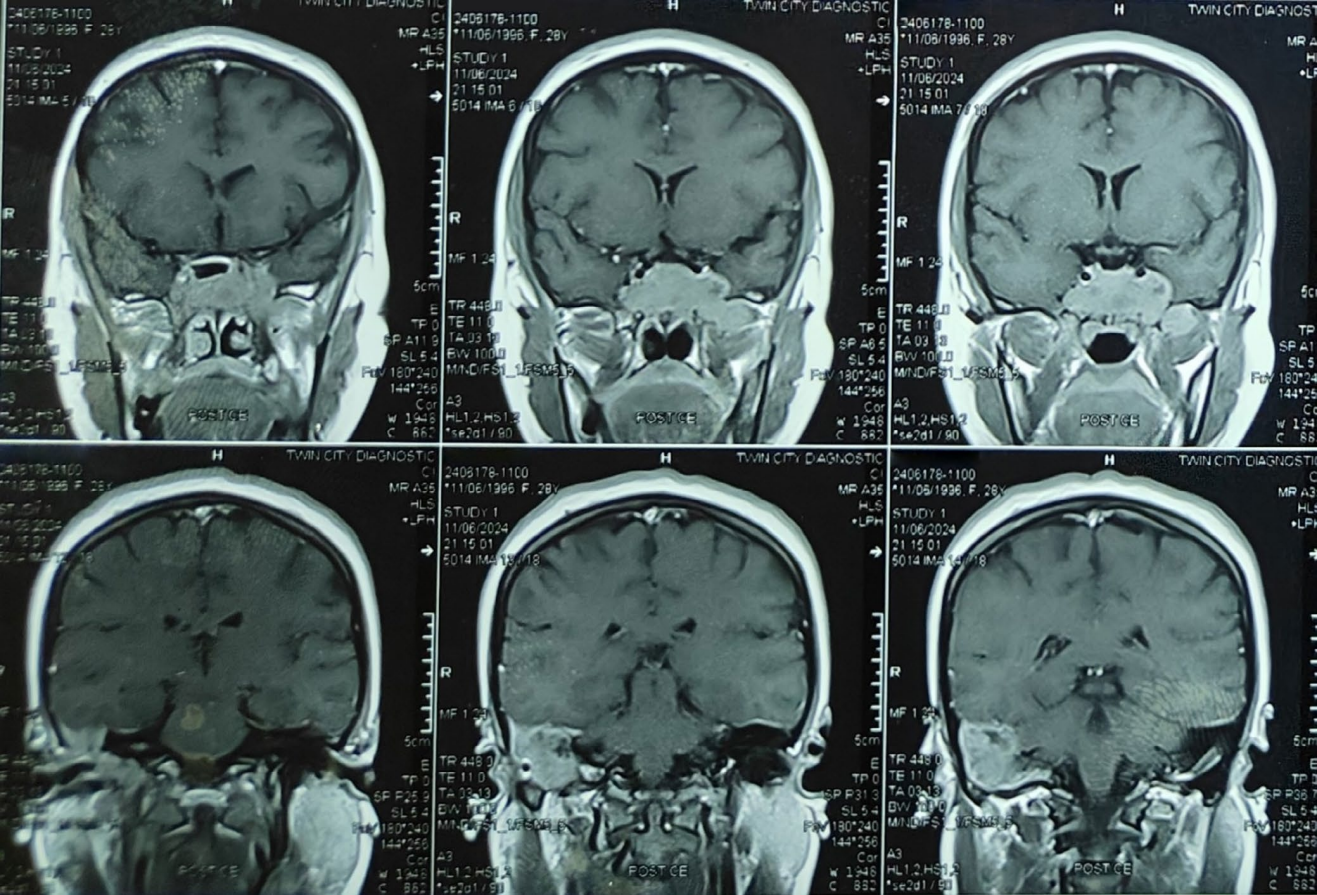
Magnetic resonance imaging: Invasion of sphenoid mass into adjacent cranial structures.
